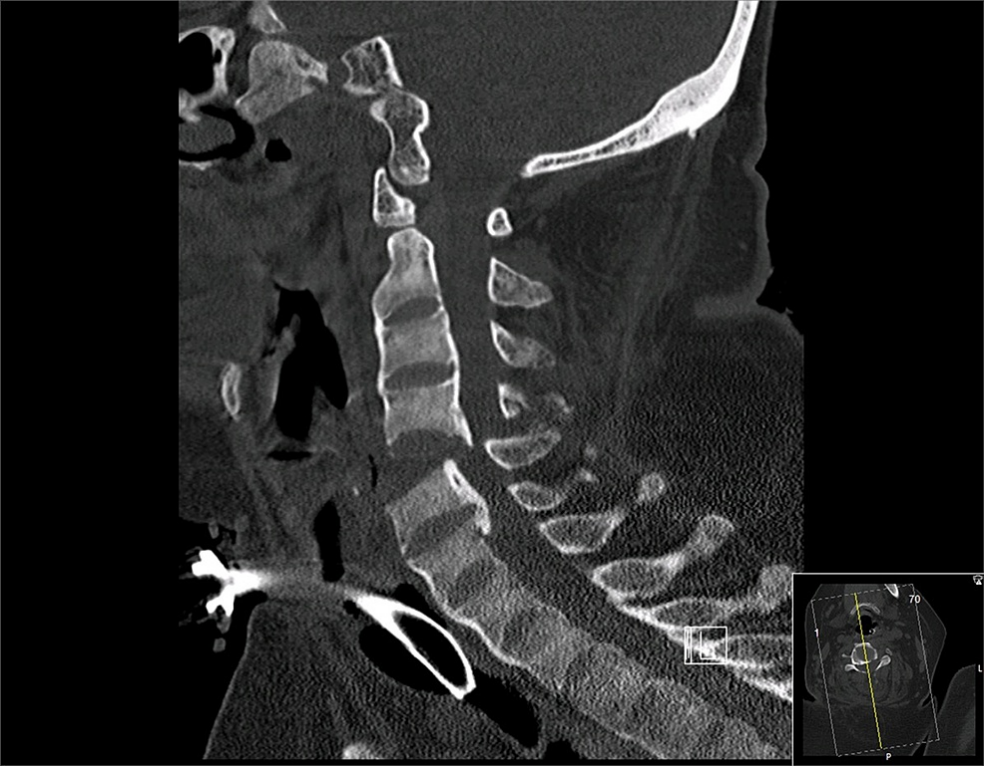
Sagittal cervical CT scan revealing fracture-dislocation at C4-5 and underlying evidence of ankylosing spondylitis and focal ossification of the posterior longitudinal ligament
